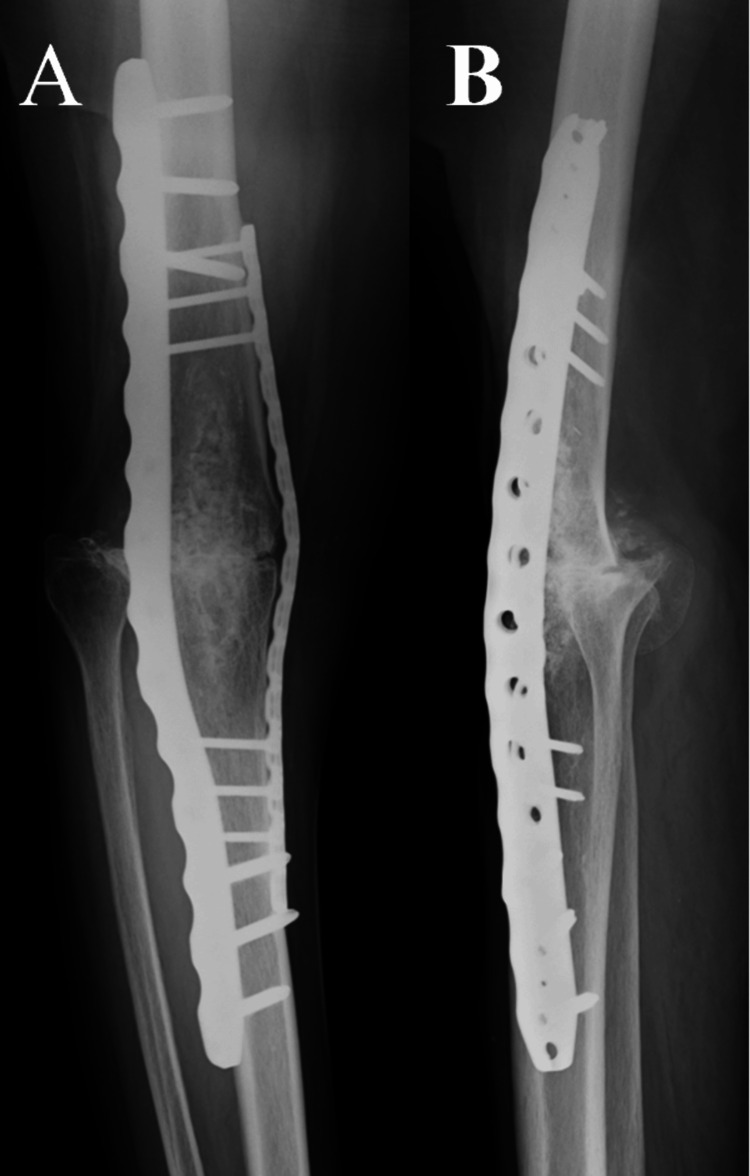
X-ray at 26 months postoperative (A) Anteroposterior radiograph of the knee joint. (B) Lateral radiograph of the knee joint.
